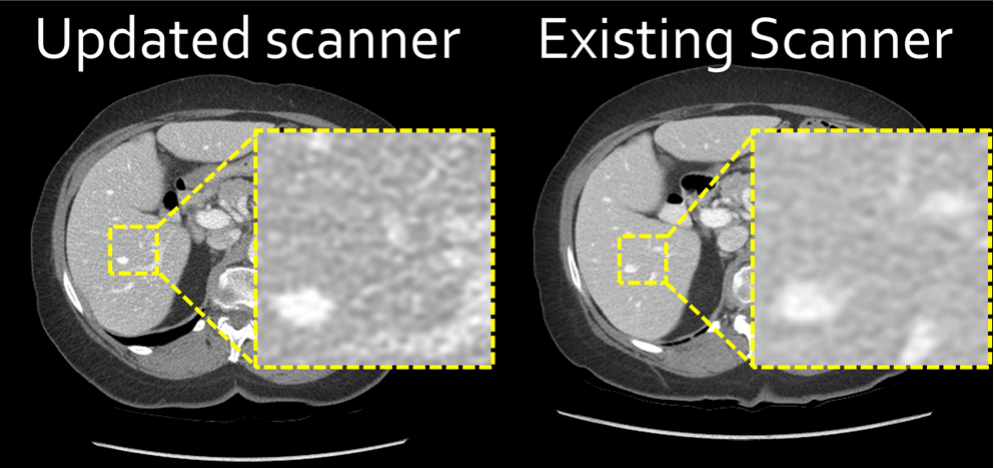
Despite using identically named standard reconstruction algorithms, the updated computed tomography (CT) scanner produced images (left) with a difference in noise appearance compared to the existing CT scanner (right), which impeded reading of body CT scans.
